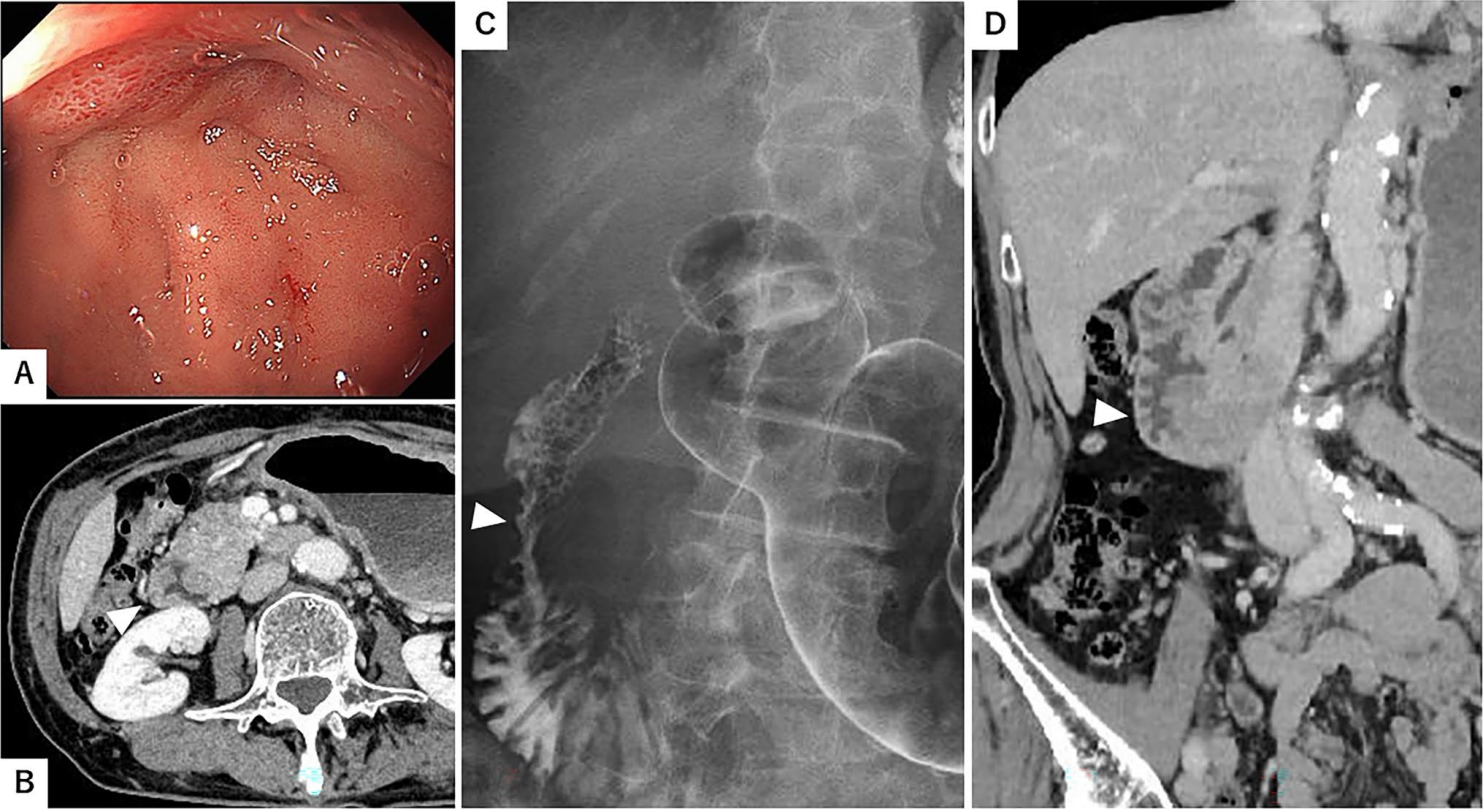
Esophagogastroduodenoscopy showed circumferential stenosis from the bulb of the duodenum that did not permit passage of the scope (A). The computed tomography scan showed thickening of the circumferential duodenal wall (B, D, triangle). Upper gastrointestinal series showed circumferential stenosis in the descending part of the duodenum (C, triangle)
